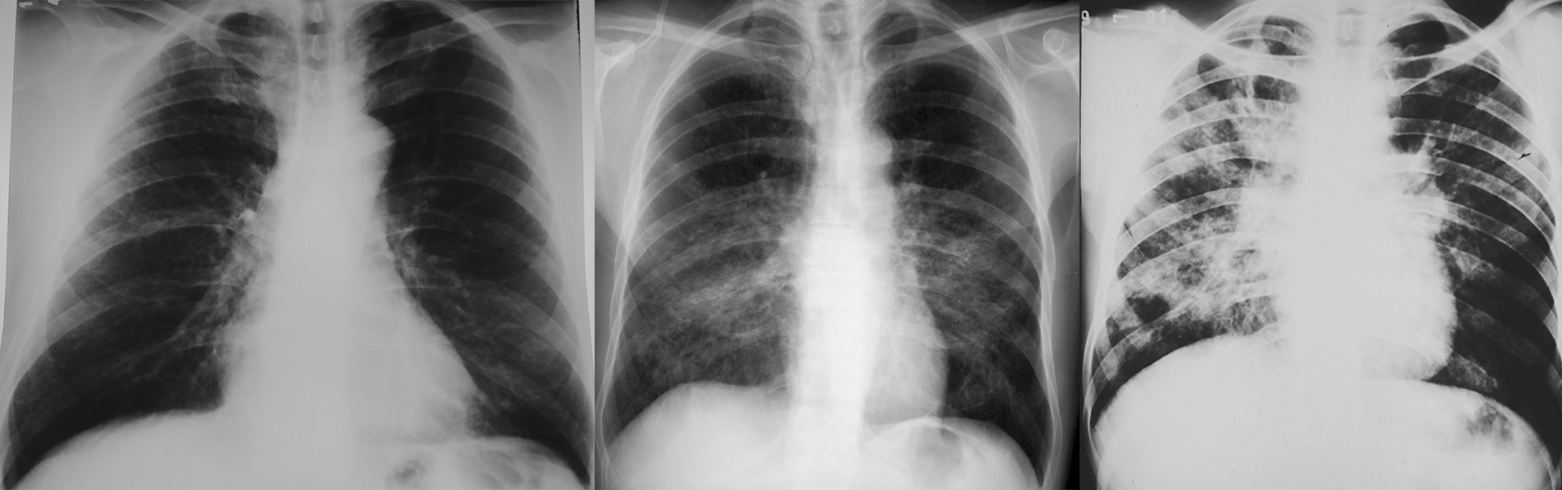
Chest roentgenograms of three cases. From left to right: mild, moderate, and severe.
